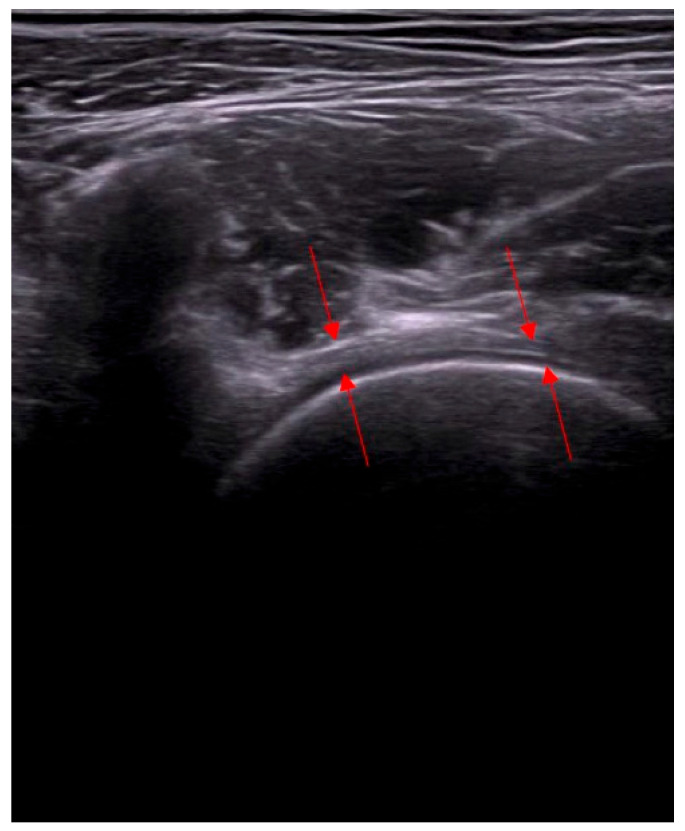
Ultrasound cross-section examination of the anterior compartment of the hip joint. Capsulitis adhesive of the front joint capsular complex—significant bolding of the joint capsule. Arrows indicate thickening of the capsular complex.
